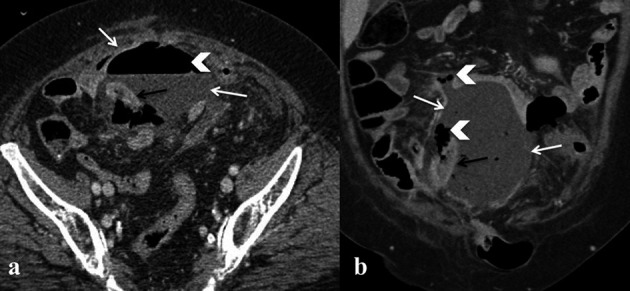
Bowel injury in a Patient who had undergone chemotherapy and cytoreductive surgery. a-b) axial and coronal Contrast-enhanced CT images show a massive abscess (white arrow) containing air (white arrowhead) and enteric material surrounding a small bowel loop (black arrow).
